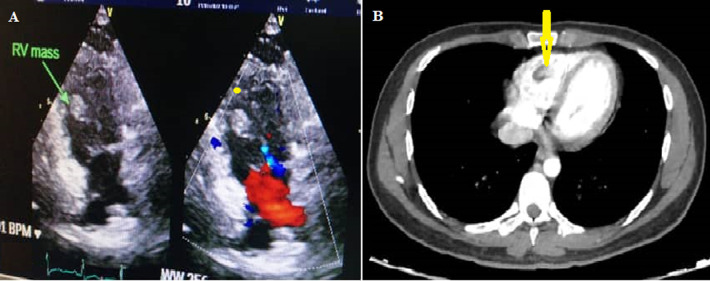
A) echocardiography showing the right ventricle mass (arrow); B) chest computed tomography scan showing the right ventricle mass (1.9 x 1 x 0.5 cm) (arrow)
